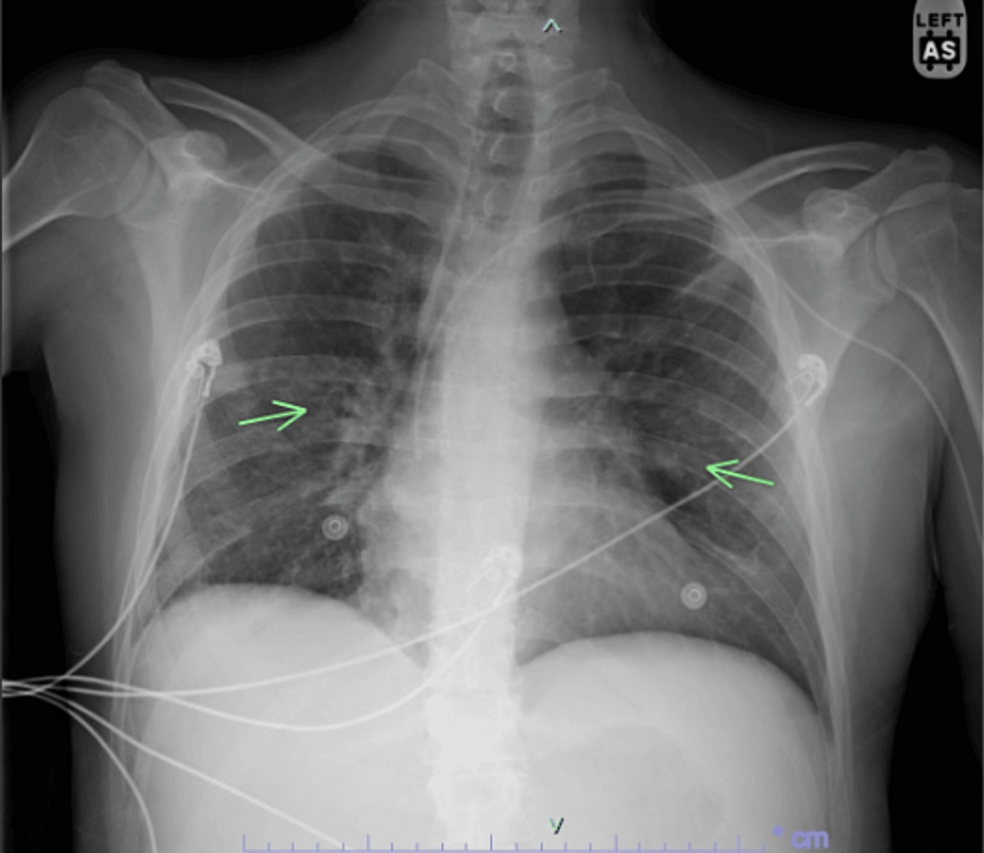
Initial chest X-ray showing residual mild interstitial prominence bilaterally, as indicated by the arrows.
